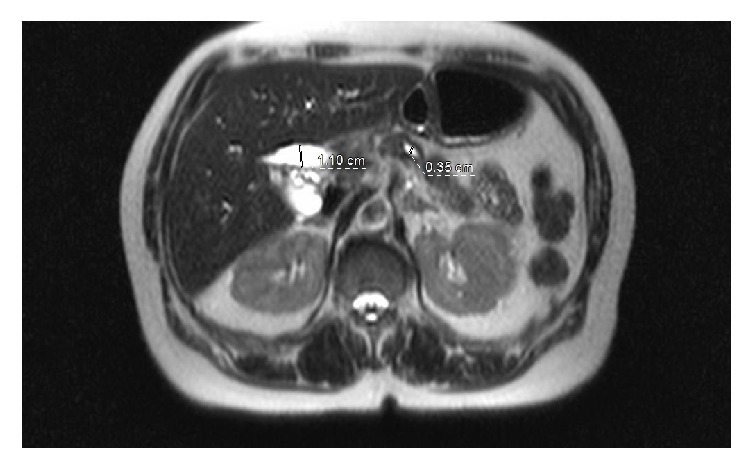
T2 axial CT: 10 mm dilated common bile duct with distended gallbladder and 3.5 mm dilated pancreatic duct.
